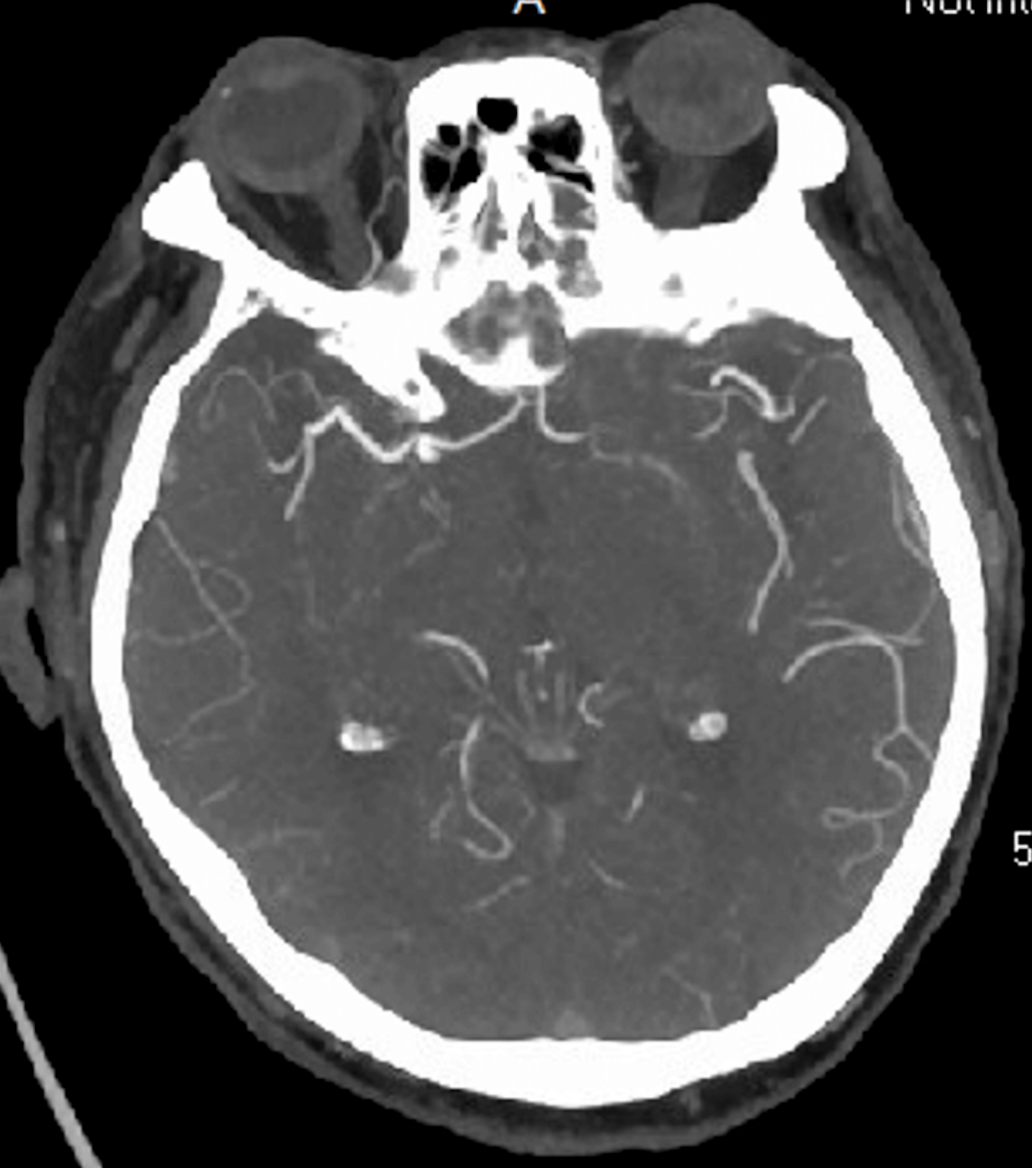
CT angiogram of the head revealing no evidence of vascular abnormalities
